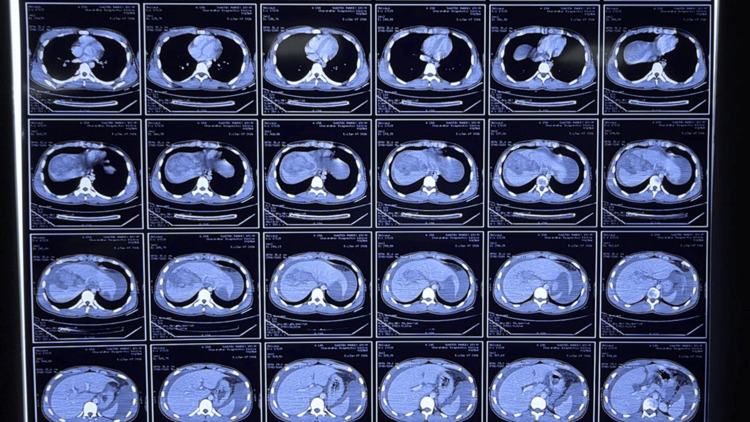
CECT whole abdomen depicting grade III liver injury with perihepatic and perisplenic collections following blunt trauma to abdomen. CECT: contrast-enhanced CT.
